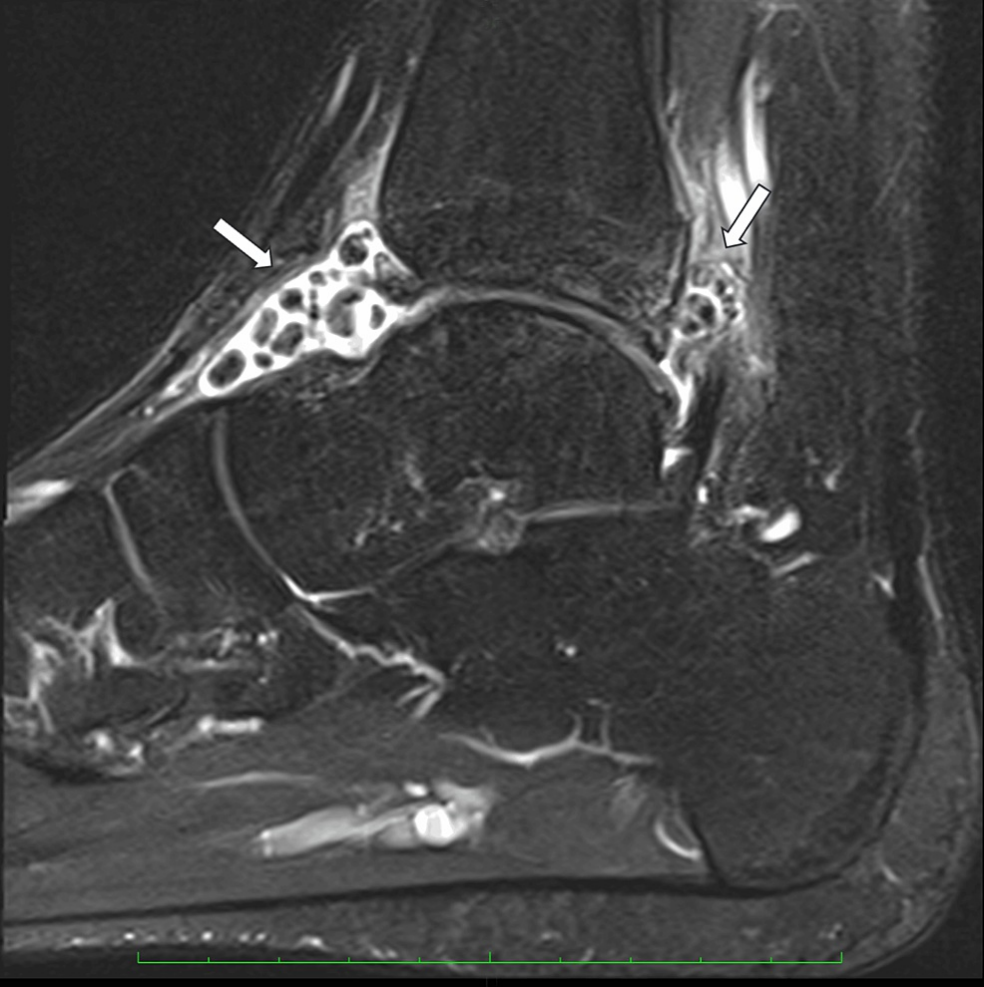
Preoperative sagittal T2 MRI of the right ankle.
